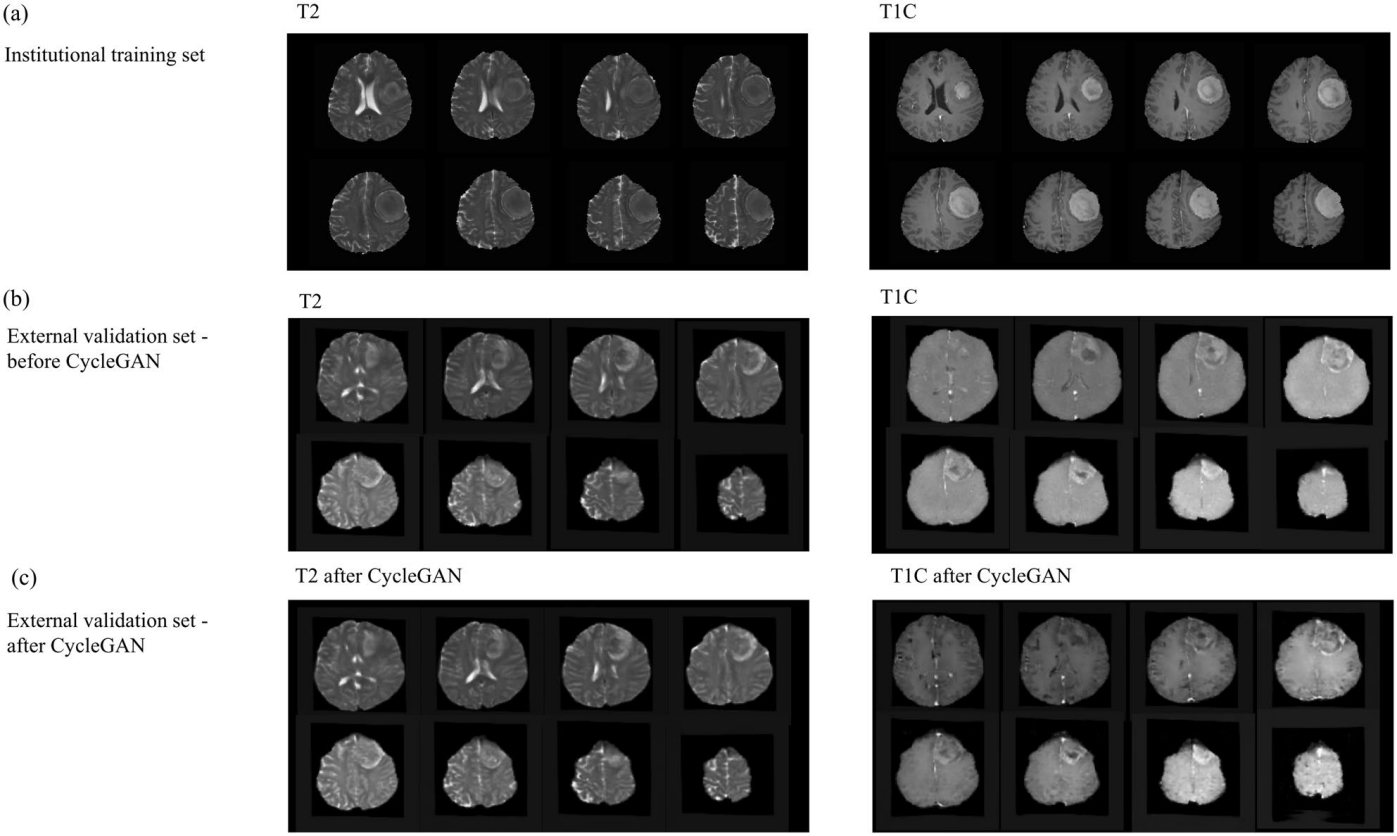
Representative cases showing the institutional training dataset and images before and after style transfer of the external validation set. (a) T2 and T1C images of a patient from the institutional training dataset that were leveraged to generate the radiomics model. (b) T2 and T1C images from the original external validation. (c) Style of T2 and T1C images from the external validation set after transformation to match that of the institutional training set images by using CycleGAN. CycleGAN = Cycle-Consistent Adversarial Networks, T1C = postcontrast T1-weighted image, T2 = T2-weighted image.
